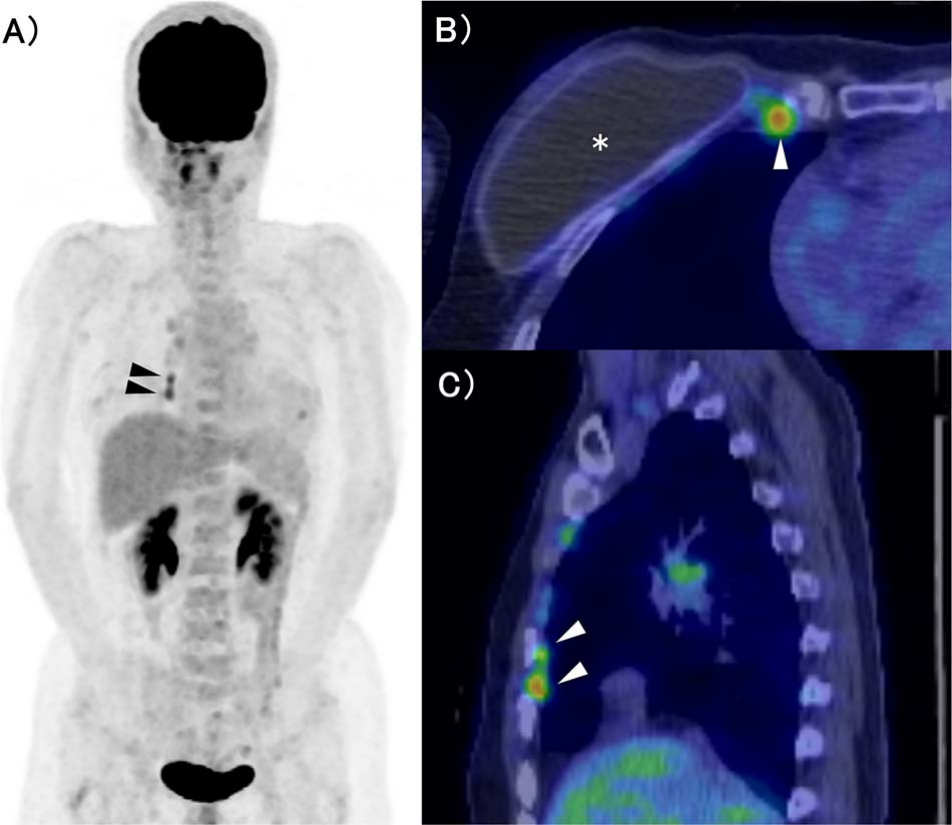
FDG-PET/CT. (A) MIP section. (B) Horizontal section. (C) Sagittal section. The asterisk and arrow indicate the artificial breast implant and IMs, respectively. FDG accumulation was observed in the IMs. FDG: Fluorodeoxyglucose, PET: Positron Emission Tomography, CT: Computed Tomography, MIP: Maximum Intensity Projection, IM: Internal Mammary lymph node.
